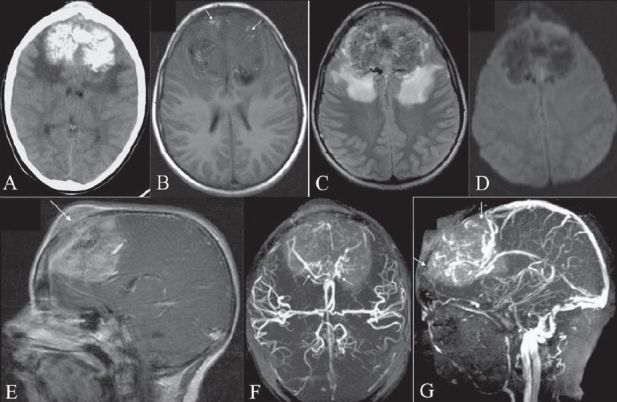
Plain CT scan (A) demonstrates an extensively calcified, hyperdense, parafalcine, extra-axial mass. Axial T1W MRI image (B) reveals the mass to be predominantly hypointense with hyperintense calcifications (arrows). An axial T2W MRI image (C) shows it to be heterogeneously isohyperintense with marked perifocal edema. An axial diffusion-weighted MRI image (D) reveals lack of restriction of diffusion within the tumor. A contrast-enhanced T1W, sagittal MRI image (E) reveals diffuse heterogeneous enhancement with transcalvarial–subgaleal extension (arrows). A three-dimensional time-of-flight MRI angiography image (F) reveals extensive tumor neovascularity with splaying and posterolateral displacement of both anterior cerebral arteries (arrows). An MRI venography image (G) demonstrates thrombosis/invasion of the anterior one-third of the superior sagittal sinus (arrow)
